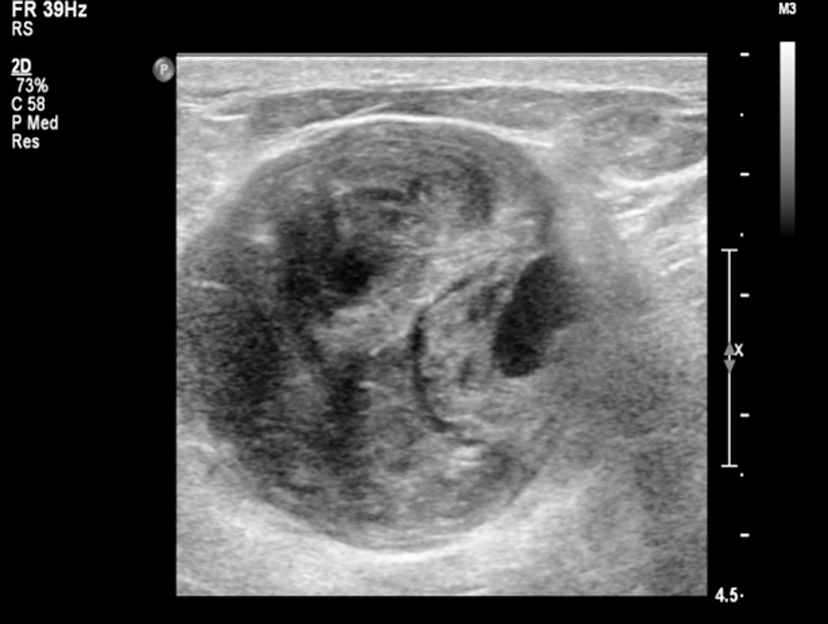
Conventional ultrasound of right breast pseudoaneurysm combined with hematoma.
